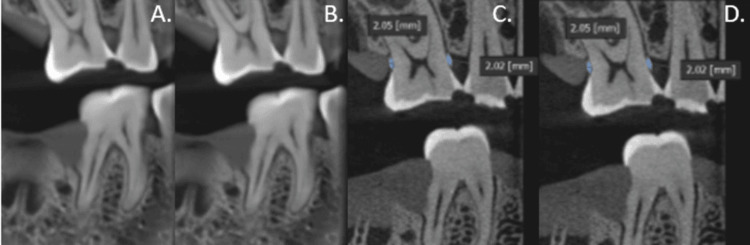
Cone beam computed tomography (CBCT) images showing carious defects at 180° (A) and 360° (B); bone loss measurements at 180° (C) and 360° (D) rotations, respectively.
